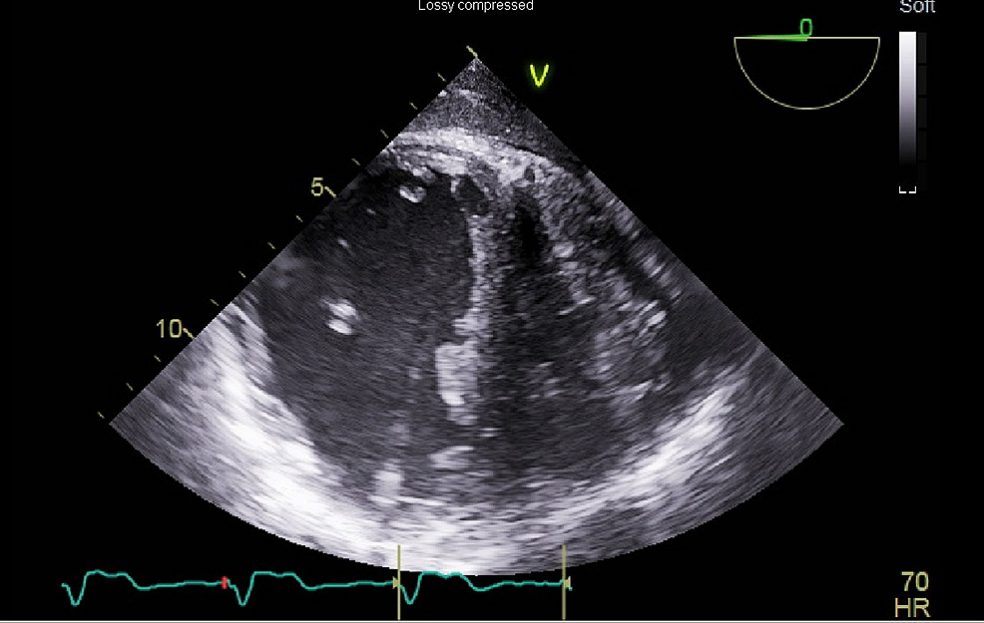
Transesophageal echocardiogram transgastric short-axis view shows severe right ventricular dilation immediately following surgical pericardial drainage.
